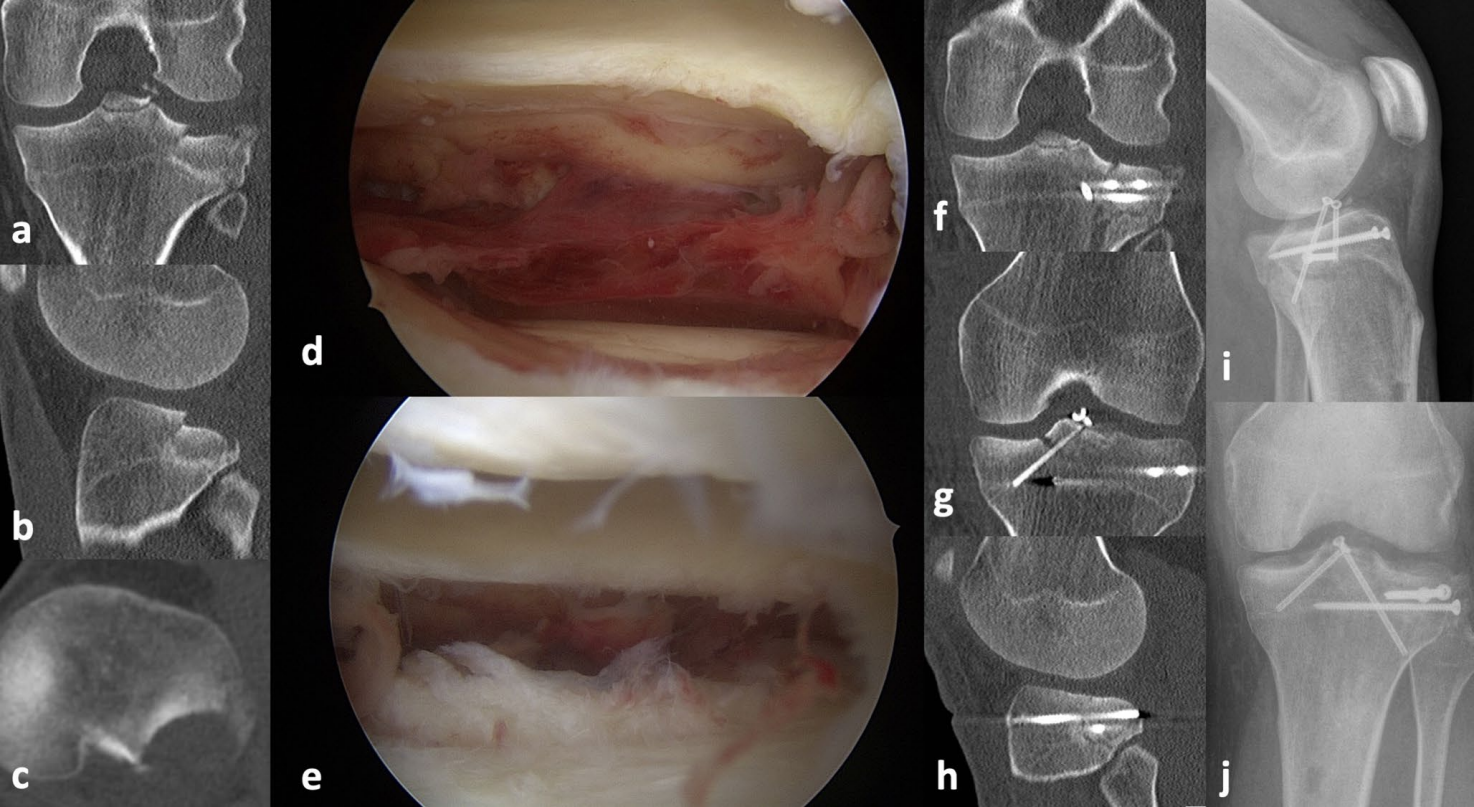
Thirty-nine-year-old patient with a Meyers-McKeever 2 eminence fracture and a Menzdorf type 2c posterolateral tibial plateau fracture (a, b, c). (d) and (e) demonstrate the initial arthroscopic view of the fracture and after reduction. (f, g and h) show the postoperative CT and (i) and (j) the final follow-up with complete bone healing
